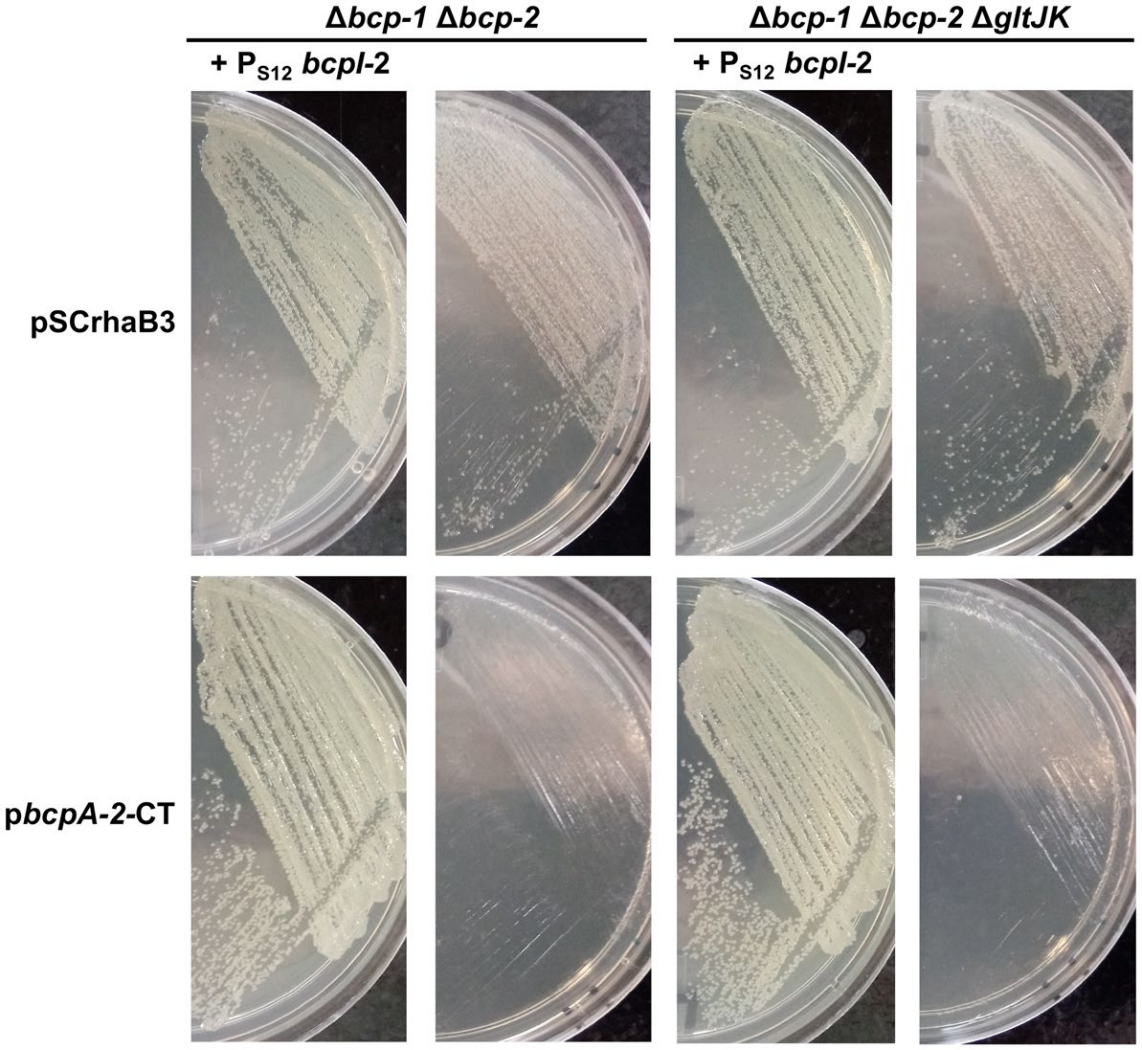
Role of *gltJK* in BcpA‐2‐CT intracellular toxicity. Transconjugants obtained after introduction of pSCrhaB3 empty vector (top) or plasmid carrying *bcpA‐2‐CT* (bottom) into Δ*bcp‐1* Δ*bcp‐2* mutant bacteria (left two columns) or Δ*bcp‐1* Δ*bcp‐2* Δ*gltJK* mutant bacteria (right two columns) that constitutively express cognate *bcpI‐2* from an *att*Tn7 site (first and third columns) or lack immunity (second and fourth columns)
